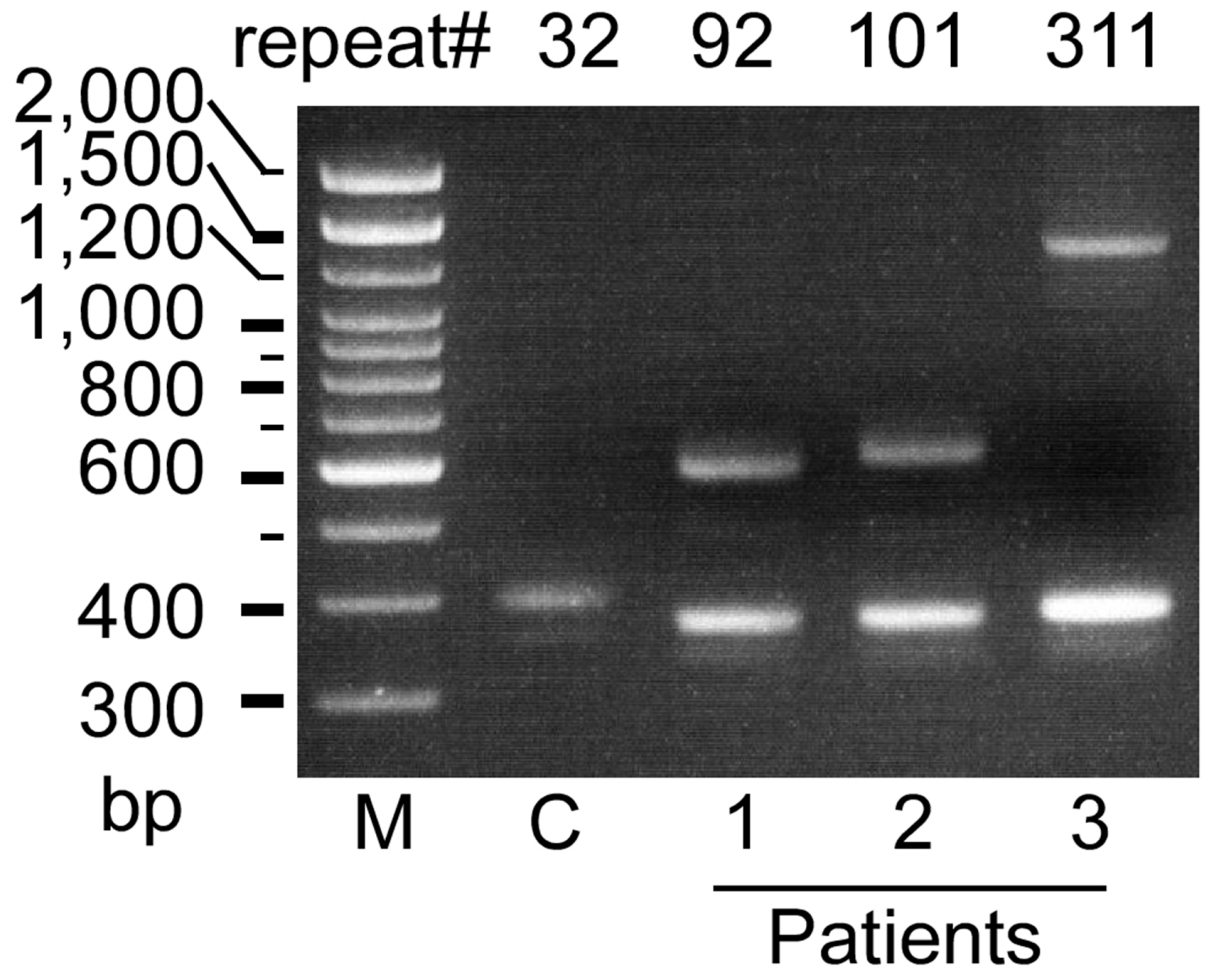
Agarose gel electrophoretic analysis of the *ATXN8OS* gene in patients with Parkinson’s disease (Pt 1–3). Three patients had expansions of the CTA/CTG repeat as indicated, whereas controls (C) had a normal repeat size. M, 100 base pair (bp) marker.
